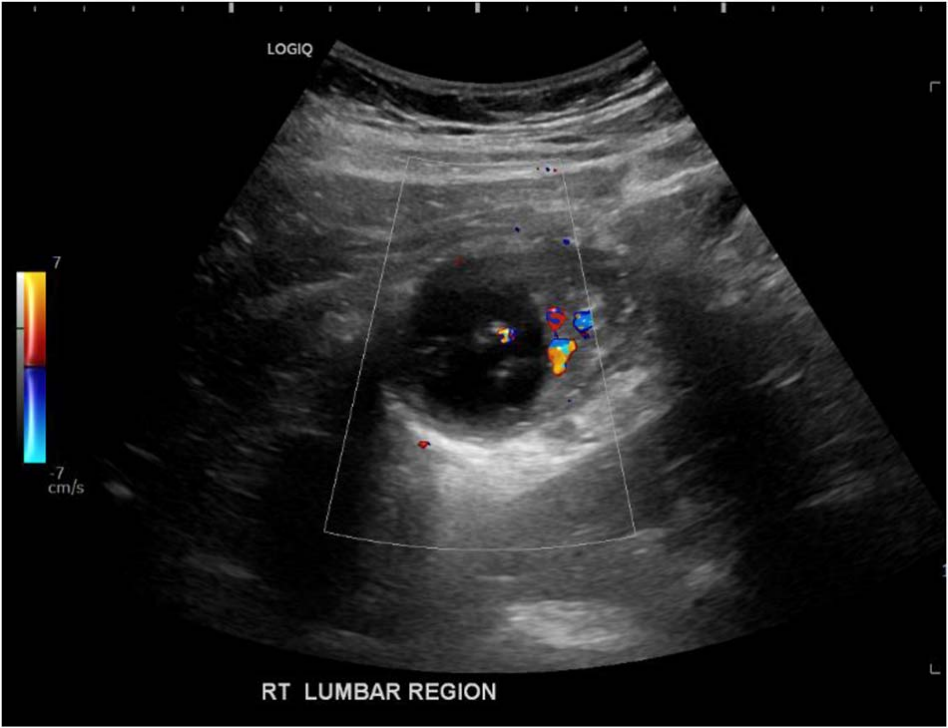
Ultrasound image demonstrating a cystic lesion with internal calcification and no hypervascularity.
